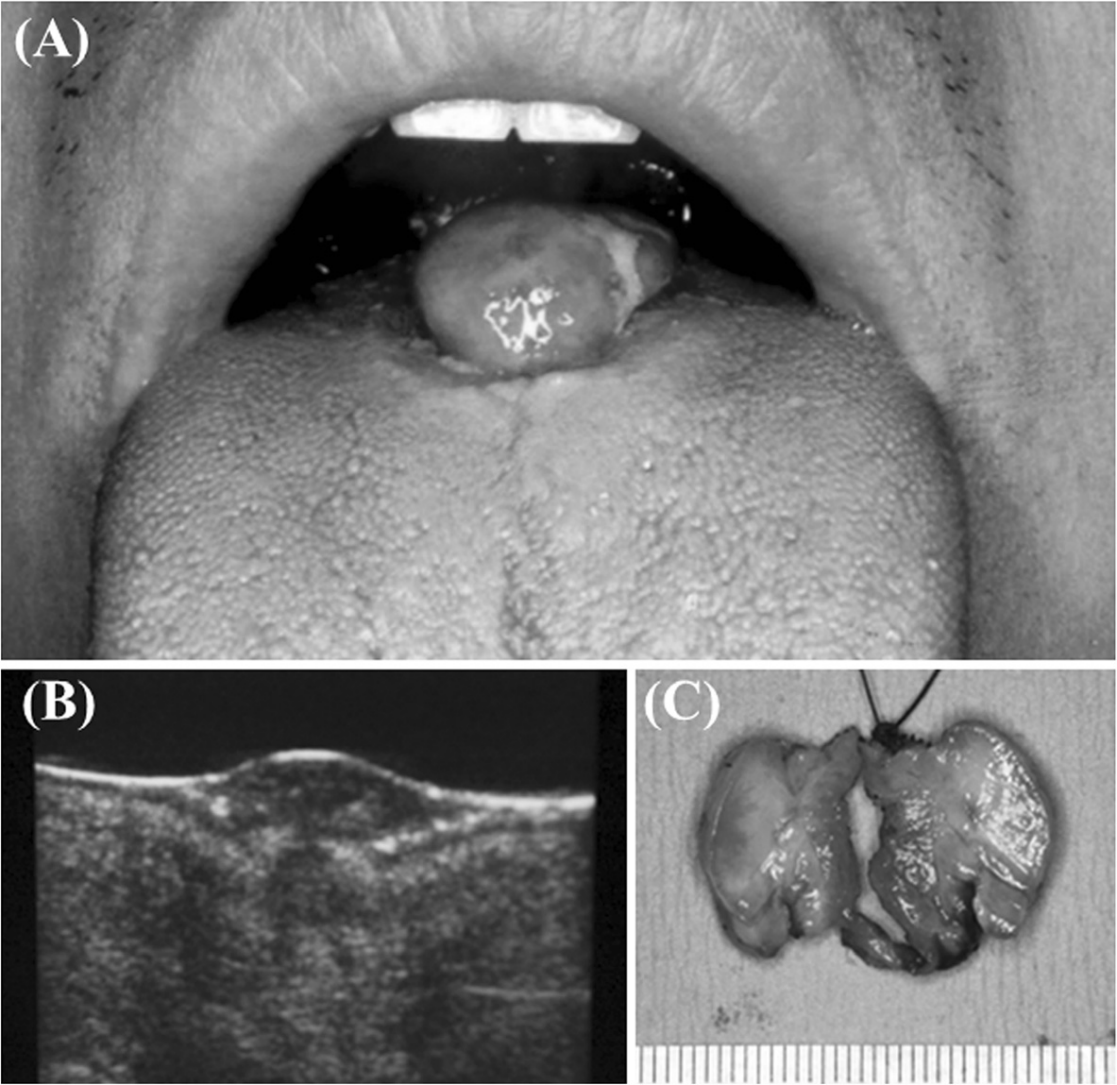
Findings of the mass in the tongue. A: Progressive swelling mass was founded at the tongue. B: Ultrasonographic findings showing a soft tissue mass. C: The cut section surface of the tumor was gray- white, solid, and firm in consistency.
